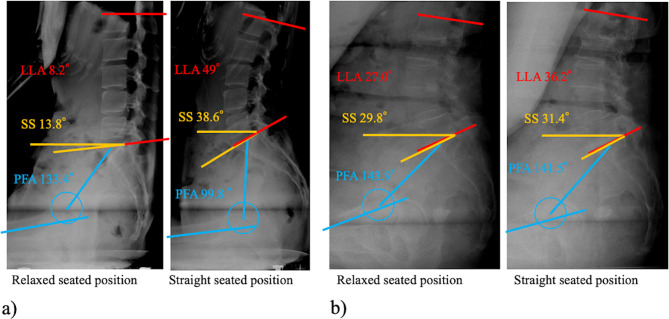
Typical radiographs showing quite the opposite change in spinopelvic sagittal alignments. (a) A 46-year-old female with a large change in sacral slope. Features: posterior rotation of the pelvis and lumbar kyphosis in the relaxed seated position. (b) A 78-year-old female patient with a small change in sacral slope. Features: anterior rotation of the pelvis and lumbar lordosis in the relaxed seated position.
